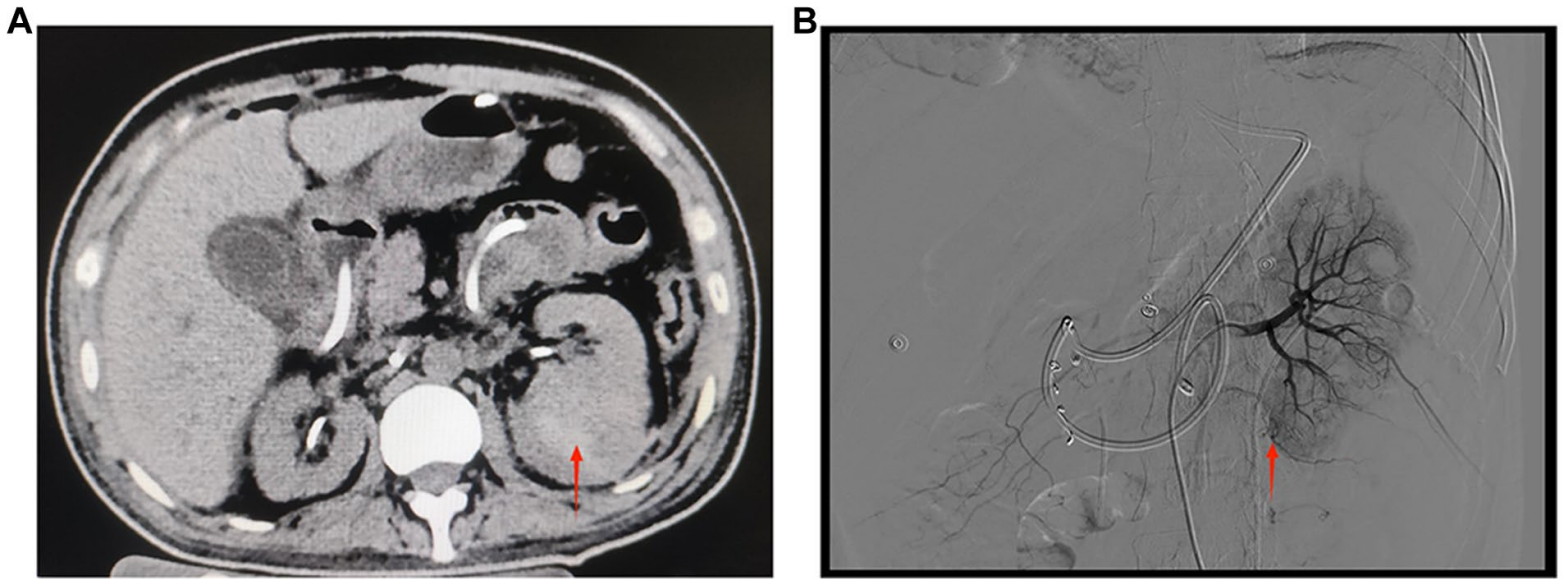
(A) CT of both kidneys. The left kidney is increased in size, heterogeneous in density, with multiple patchy hyperdense shadows and a few flocculent hyperdense shadows seen in the perirenal space of the left kidney. The red arrow indicates a hematoma in the lower pole of the left kidney (September 27, 2022, day 5 of transfer to ICU). (B) Digital subtraction angiography of the left renal artery. The red arrow shows suspicious active extravasation of the subsegmental artery supplying the lower pole (October 18, 2022, day 26 of transfer to ICU).
